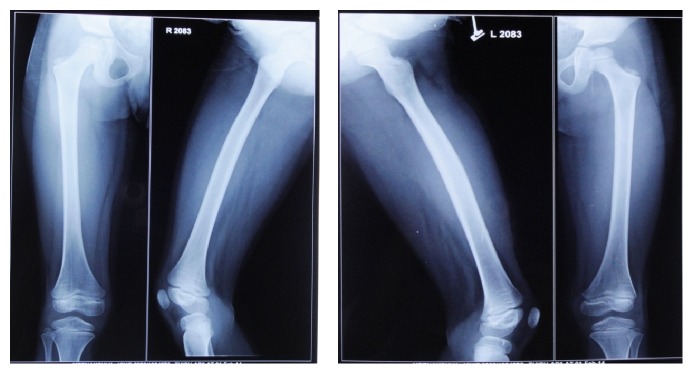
Radiograph of hip with femur and tibia.
